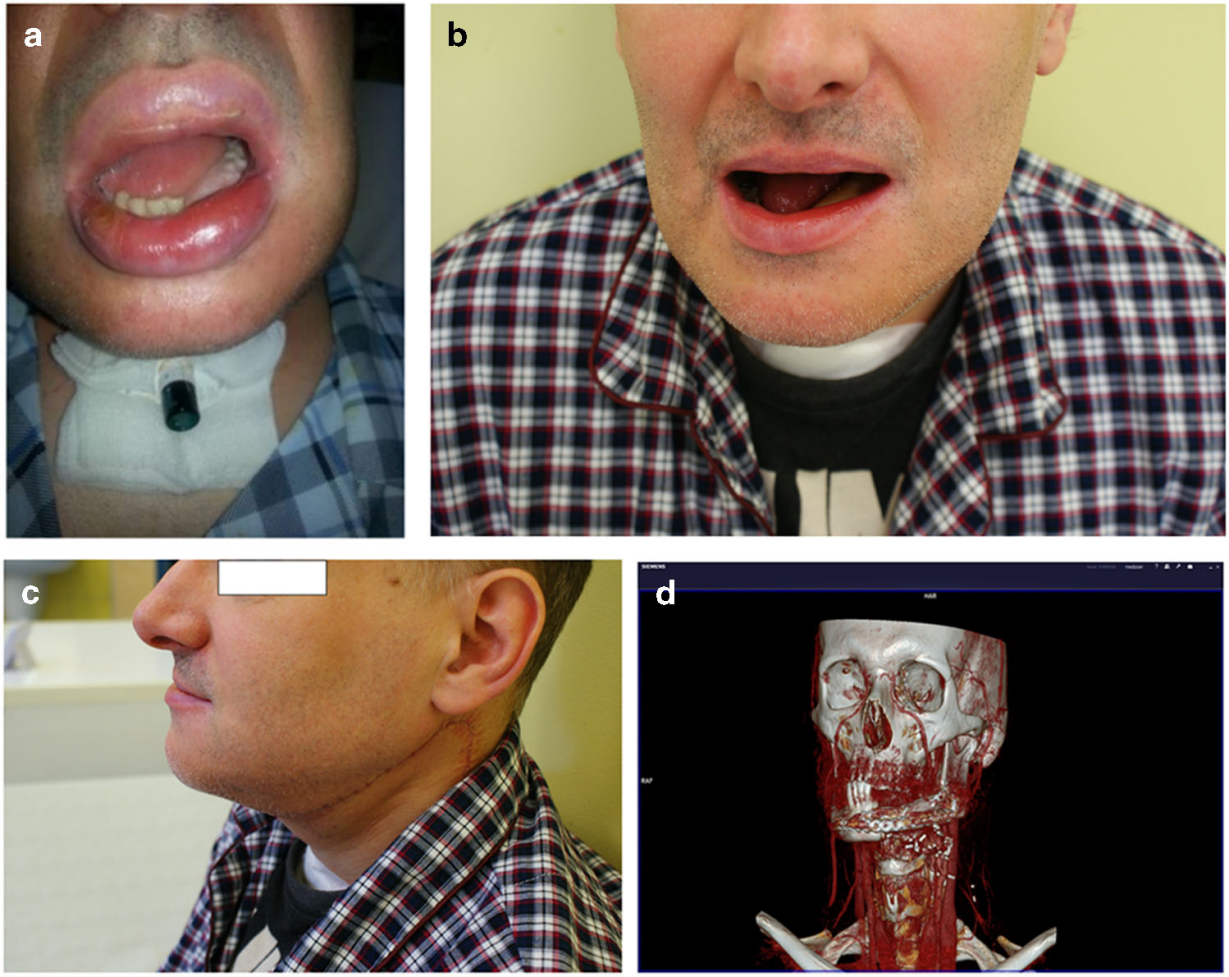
Patient following reconstructive surgery (after partial body resection of the mandible with floor of the mouth) with fibula flap using 3D model printing. a 1 week after surgery with fibula flap. b Frontal view of the patient 2 months after surgery with fibula flap. c Lateral view of the patient 2 months after surgery with fibula flap. d CT reconstruction scan
